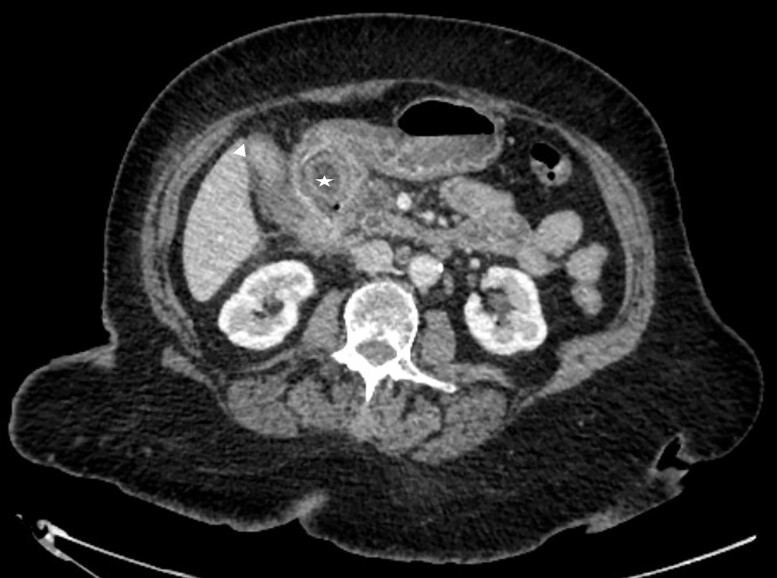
CT scan showing the stone impacted in the first part of duodenum.
